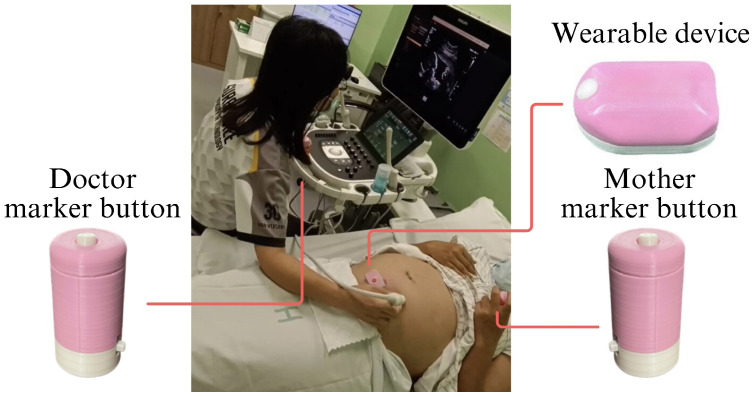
Two-stage labeling protocol for fetal movement annotation, combining maternal button press with real-time ultrasound confirmation.
